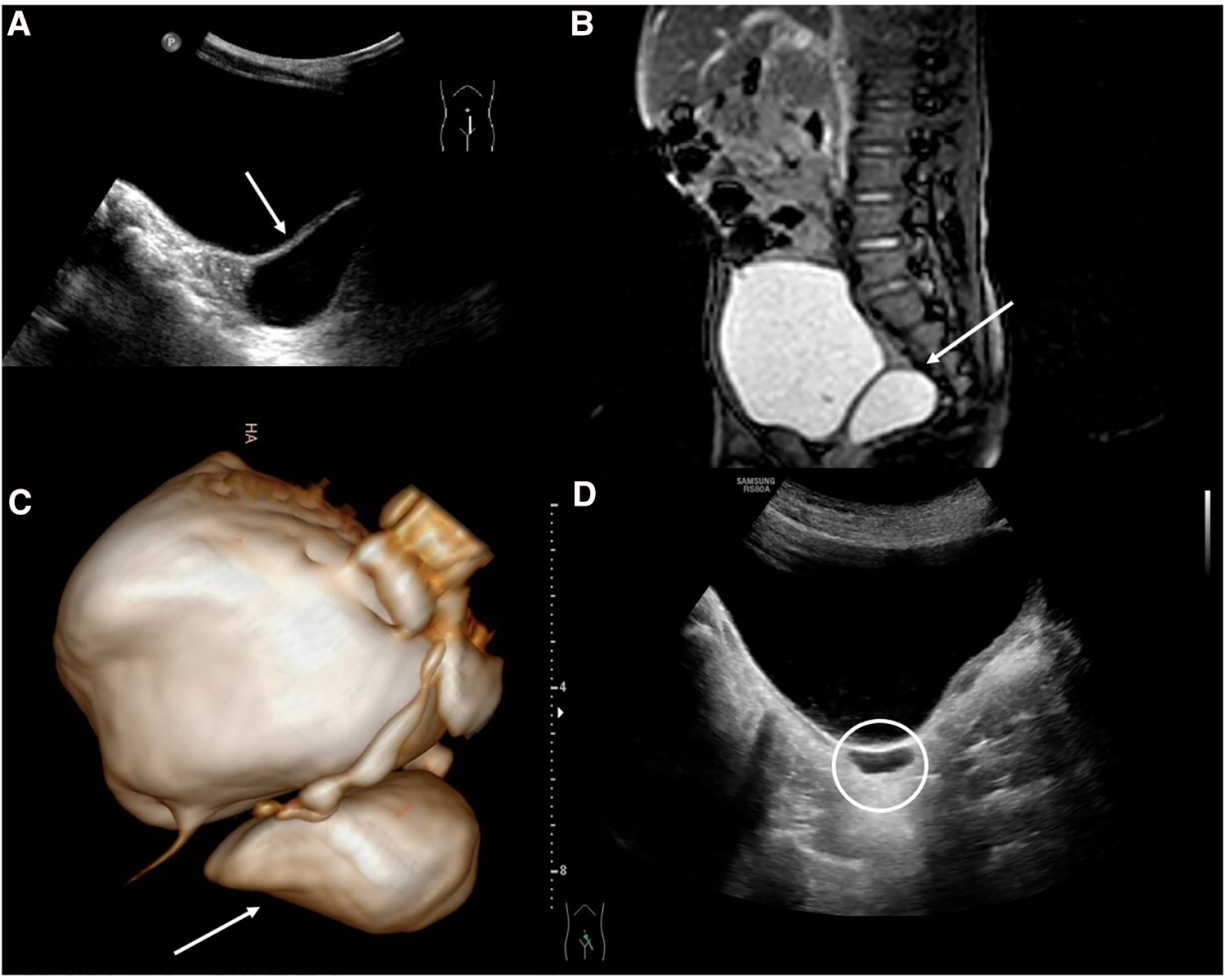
(A) US study showed a 36 mm × 16 mm right retrovesical fluid-filled cyst; (B) MRI showing the retrovesical SVC; (C) 3D MRI reconstruction; (D) postoperative US showing; the persistence of the 4 mm × 14 mm cyst.
